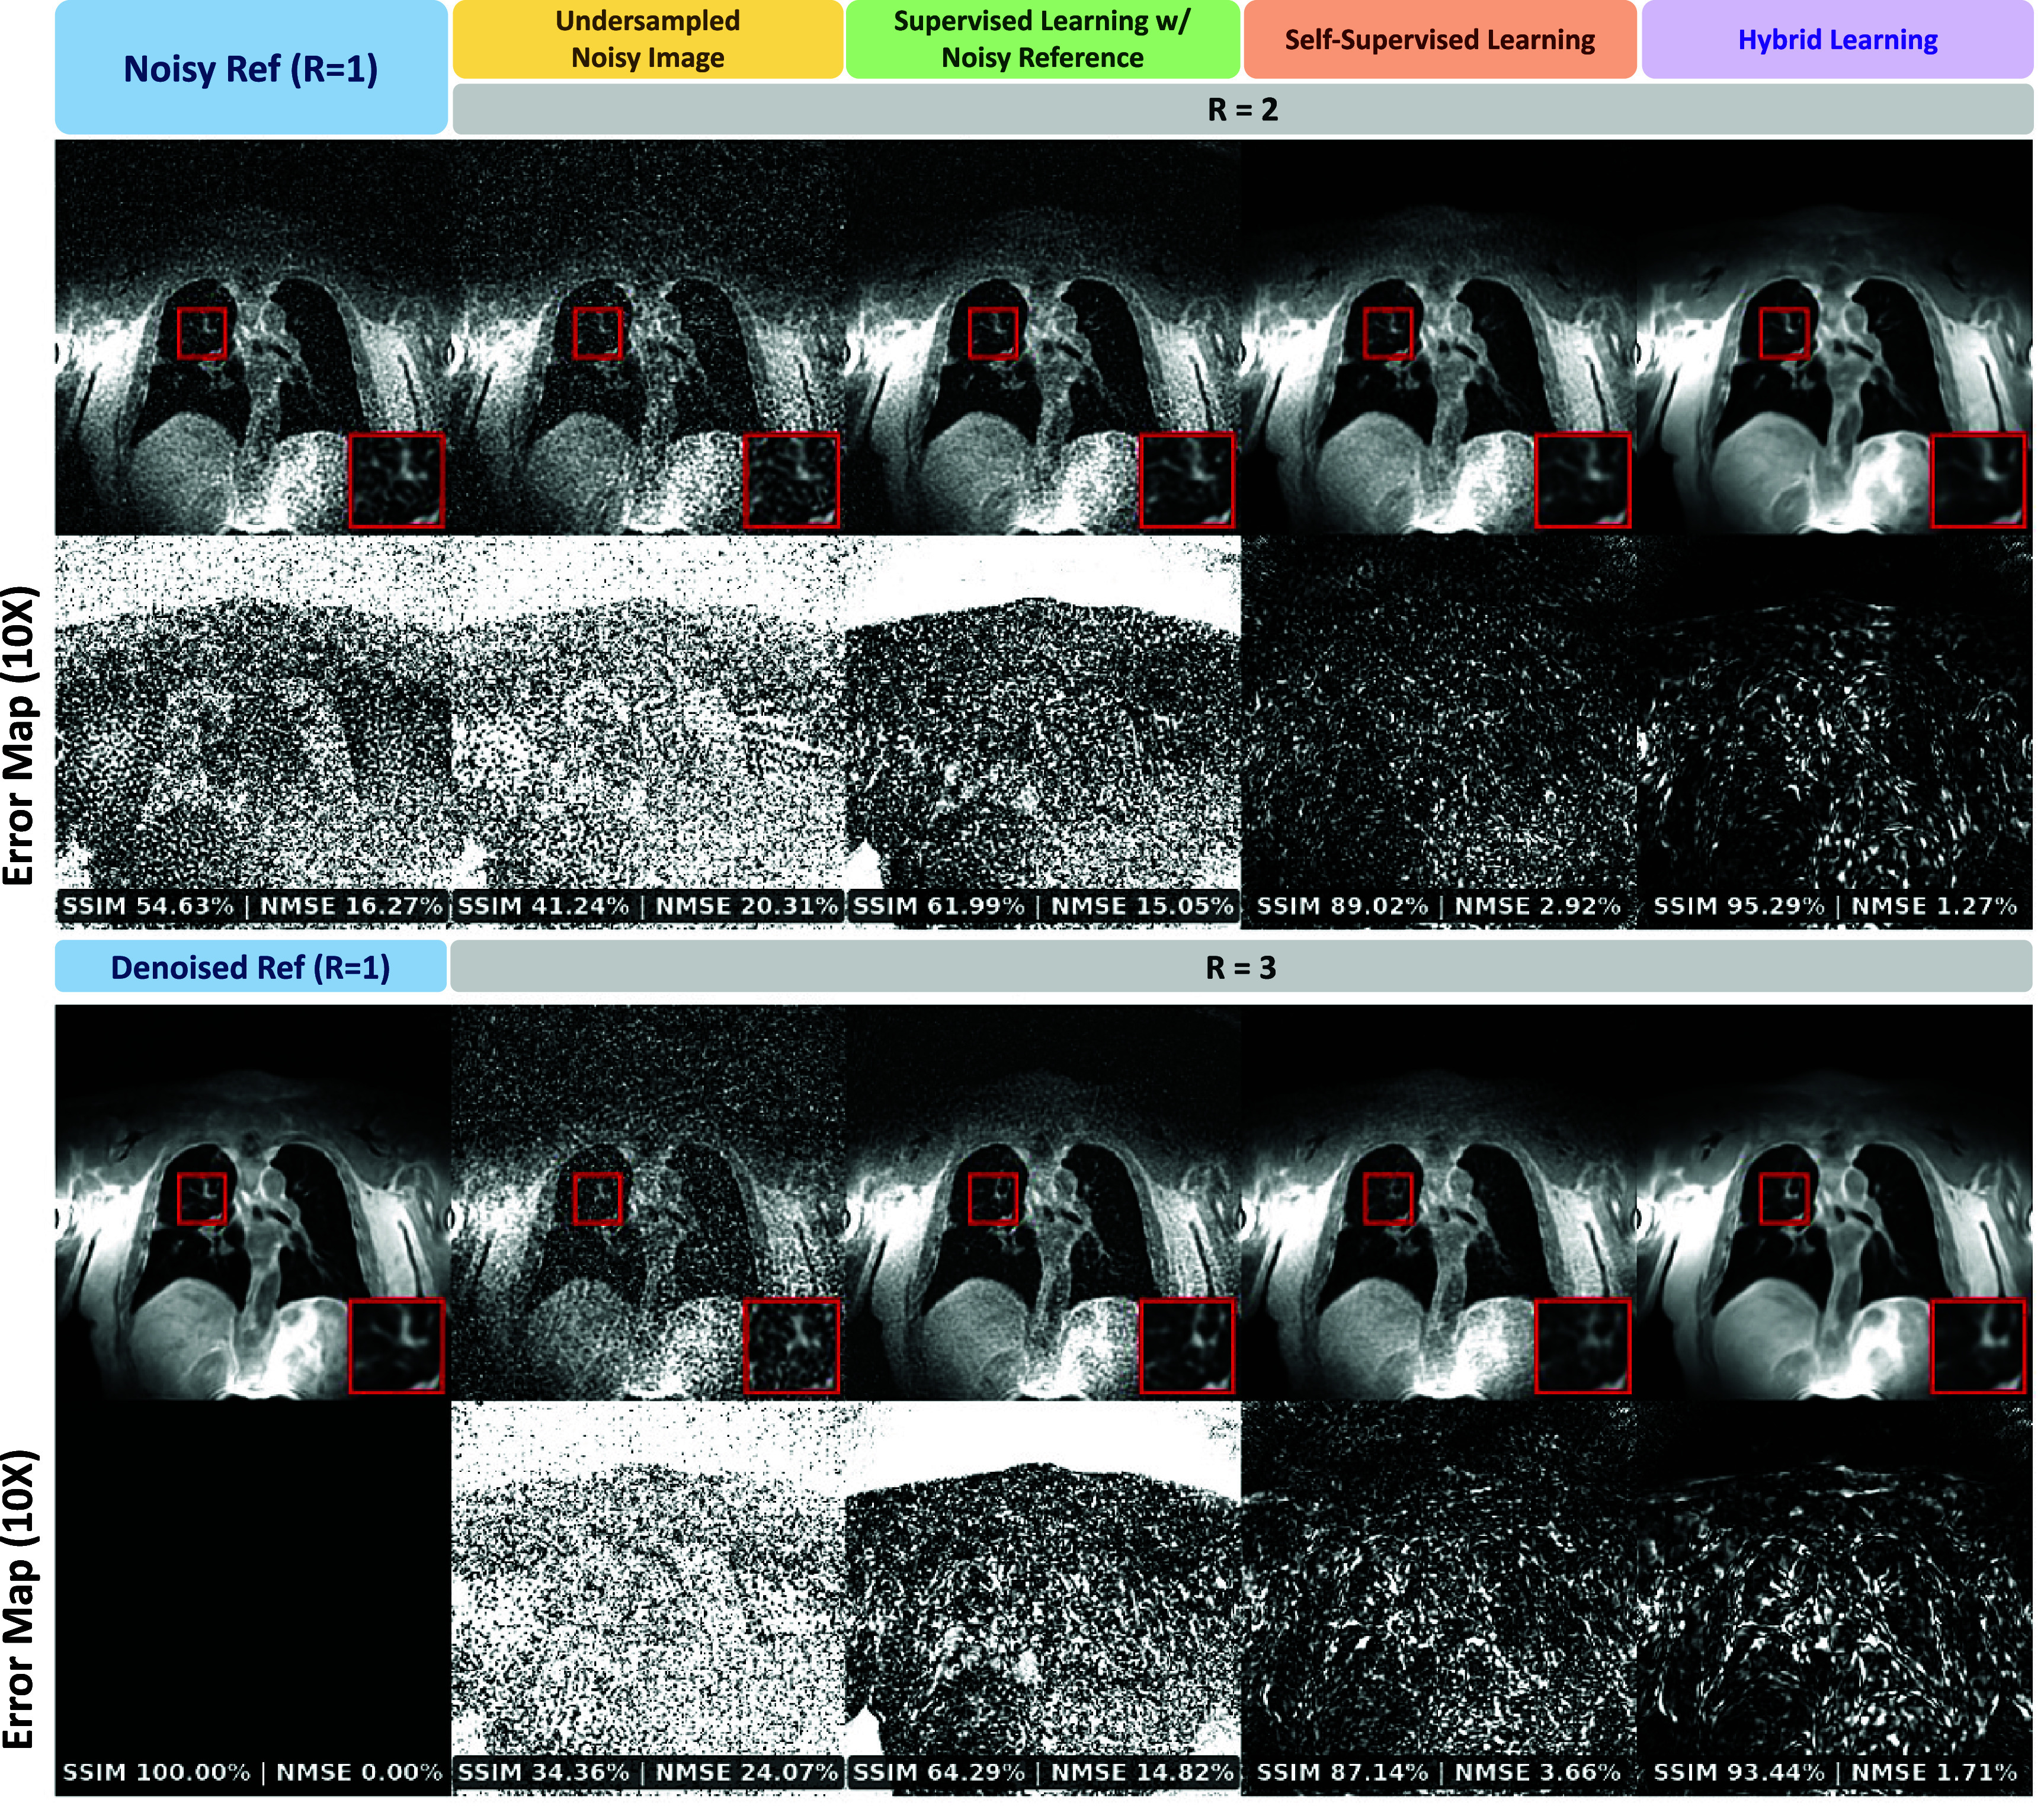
Lung MRI (0.55 T), spiral sampling at *R* = 2 and 3. Another representative case at *R* = 2 and 3 with a higher baseline noise level in the original image (Noisy Ref). Under this more challenging condition, hybrid learning still achieved improved reconstruction quality, with more effective suppression of noise and undersampling artifacts, even though the performance of all methods degraded compared with cases with a lower baseline noise level.
